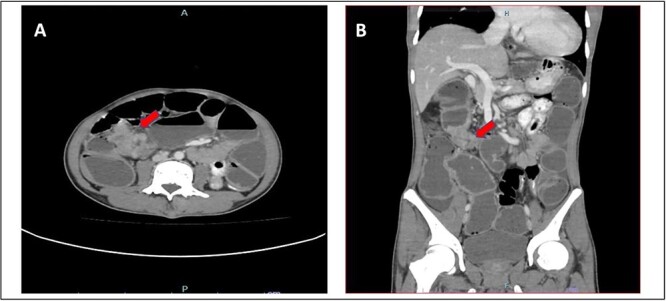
(A) Axial view and (B) coronal view of computed tomography showed short segment thickening of proximal transverse colon (red arrow) with dilatation of bowel.
